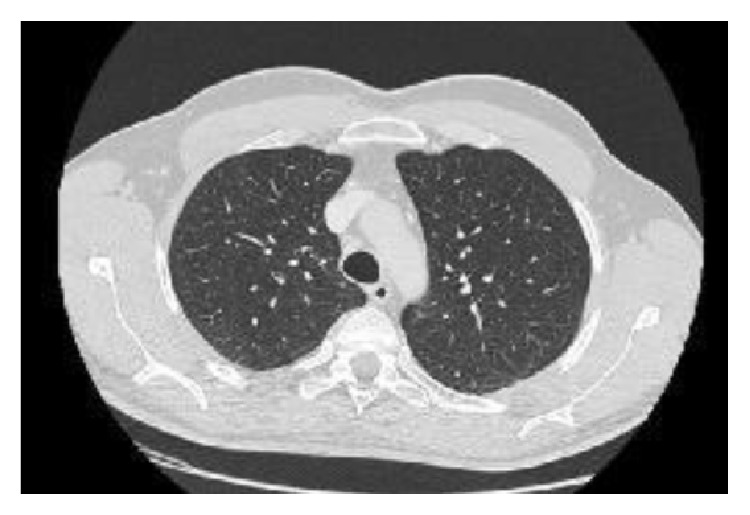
HR-CT lung pretreatment: resolution of interstitial flogistic involvement.
